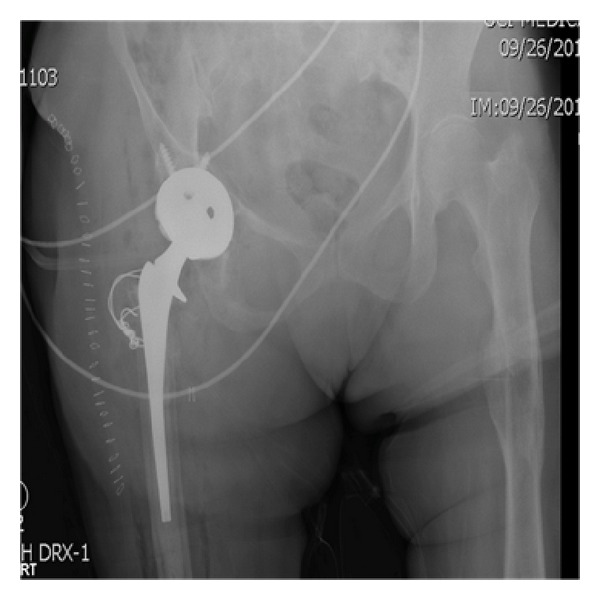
Postoperative radiograph status after reduction of dislocated hip.
